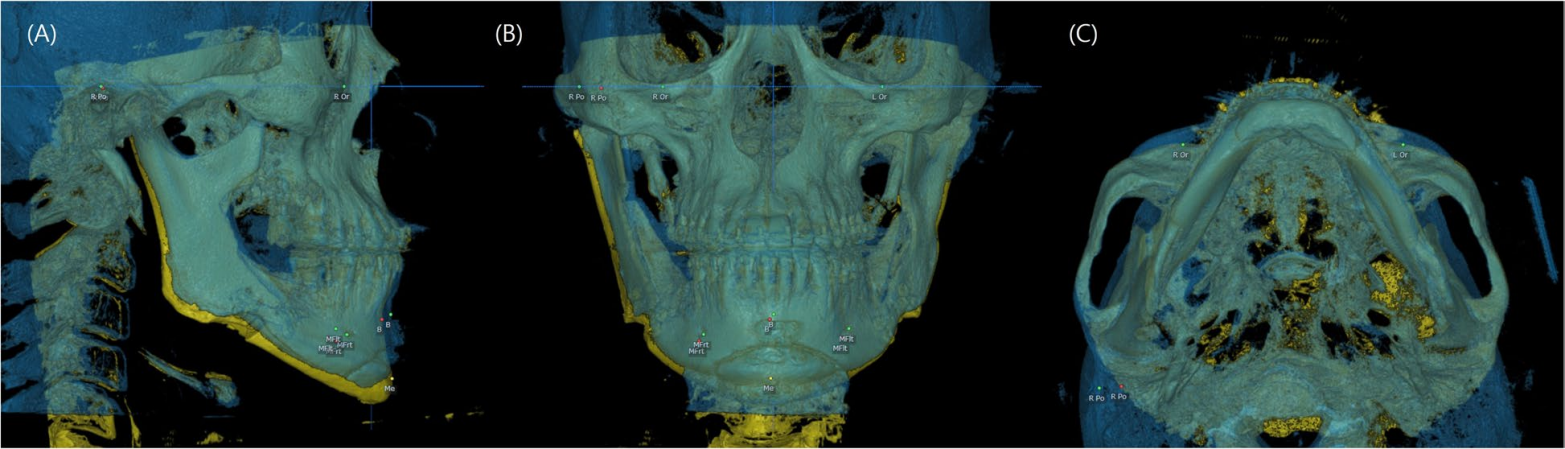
A superimposed 3D-CBCT directly after surgery (yellow) and 6–12 months after surgery (blue). The position change of the B point and mental foramen. (A): lateral view right; (B): frontal view; (c): submentovertex view
